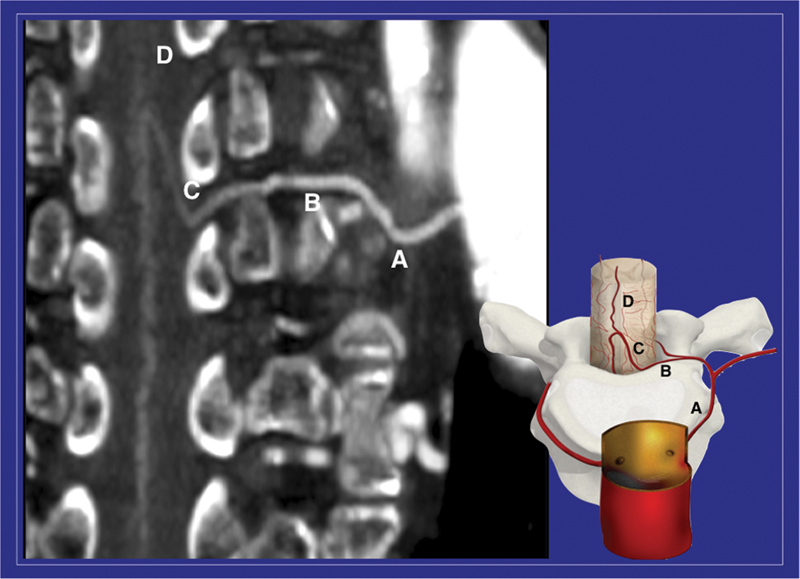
With preoperative computed tomography angiography, using postprocessing tools, the whole path of the arterial feeder to the spinal cord can be visualized, from the aorta to the anterior spinal artery (A: intercostal artery; B: anterior radicular artery; C: arteria radicularis magna or artery of Adamkiewicz; D: anterior spinal artery).
